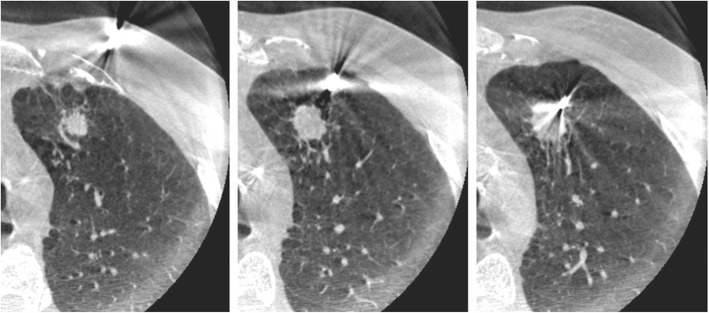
Progressive images of Cone-beam-guided chest biopsy of a pulmonary nodule in the left upper lobe
